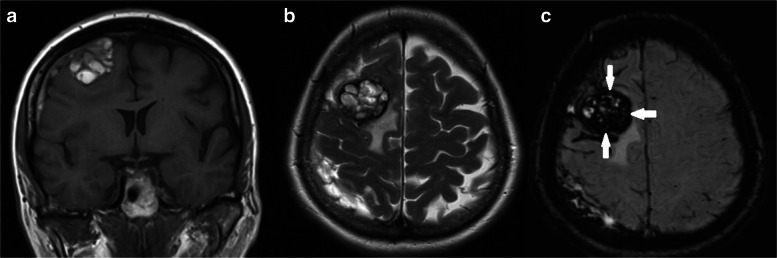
a Preoperative MRI. T1 weighted image, coronal plane. A mass sized 32 * 30 * 35 mm on the surface of the right frontal lobe, with heterogeneous iso- hyperintensive signal (lesion has «popcorn» sign), right chronic subdural hematoma with midline brainshift 3 mm. b Preoperative MRI. T2 weighted image, axial plane. This mass shows similar popcorn-shaped characteristics with perifocal edema. c Preoperative MRI. SWI sequence, axial plane. Ring of hypointensity around lesion (arrows)
